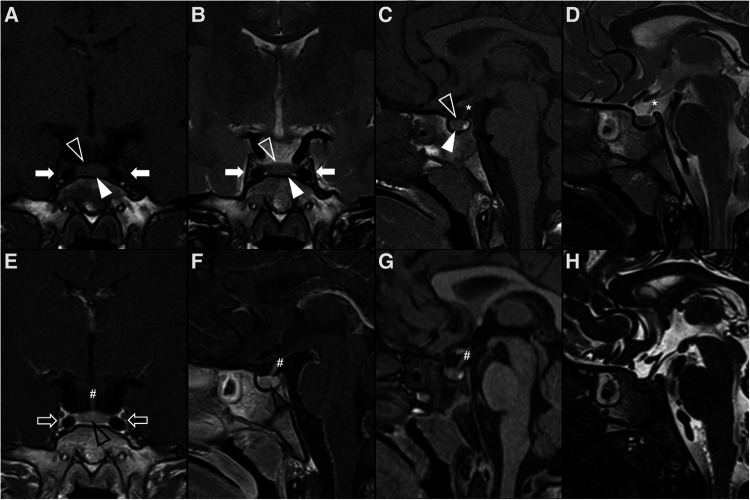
Normal appearing pituitary gland and sella in a 6-year-old girl. Brain MRI including coronal (A) and sagittal (C) T1WI as well as coronal (B) and sagittal (D) T2WI reveal a normal sized sella turcica as well as regular volume and morphology of the adenohypophysis according to age and gender (white arrowhead). Note symmetry and homogeneous signal intensity of the gland and flattened superior border (empty white arrowhead). The pituitary stalk is in the midline and presents regular thickness (asterisks). The spontaneous T1 hyperintensity of the neurohypophysis, related to vasopressin storage, is better depicted in sagittal imaging in the posterior aspect of the sella turcica (black arrowhead). Also note normal permeability of the suprasellar cistern and regular lateral concavity of the cavernous sinuses (white arrows). Coronal (E) and sagittal (F) T1WI after gadolinium injection reveal normal homogeneous enhancement of the pituitary gland (empty black arrowhead), pituitary stalk (hashtag), and cavernous sinus (empty white arrows) due to absence of blood brain barrier. MR imaging should also include pre (G) and post-contrast volumetric T1 MPRAGE imaging not shown) for morphological characterization of brain as well as better depiction of focal areas of hypo-enhancement within the pituitary gland or characterization of other sellar/suprasellar masses, respectively. Sagittal 3D T2-weighted sequences (e.g., CISS) (H) are useful in the anatomical characterization of the sellar region including pituitary gland as well as other midline structures and cystic lesions.
